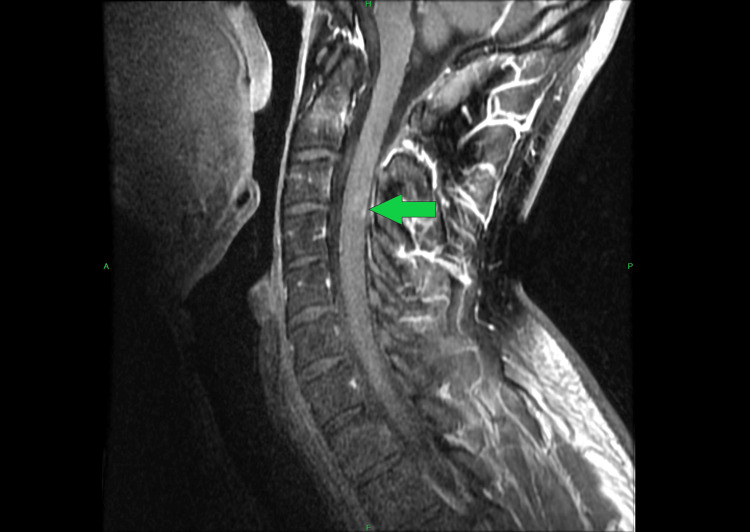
Cervical Spine 3 Tesla MRI Sagittal View With Intravenous Contrast On the sagittal postcontrast images, there was suggestion of minimal specks of enhancement noted at the more inferior aspect of the lesion (green arrow).
